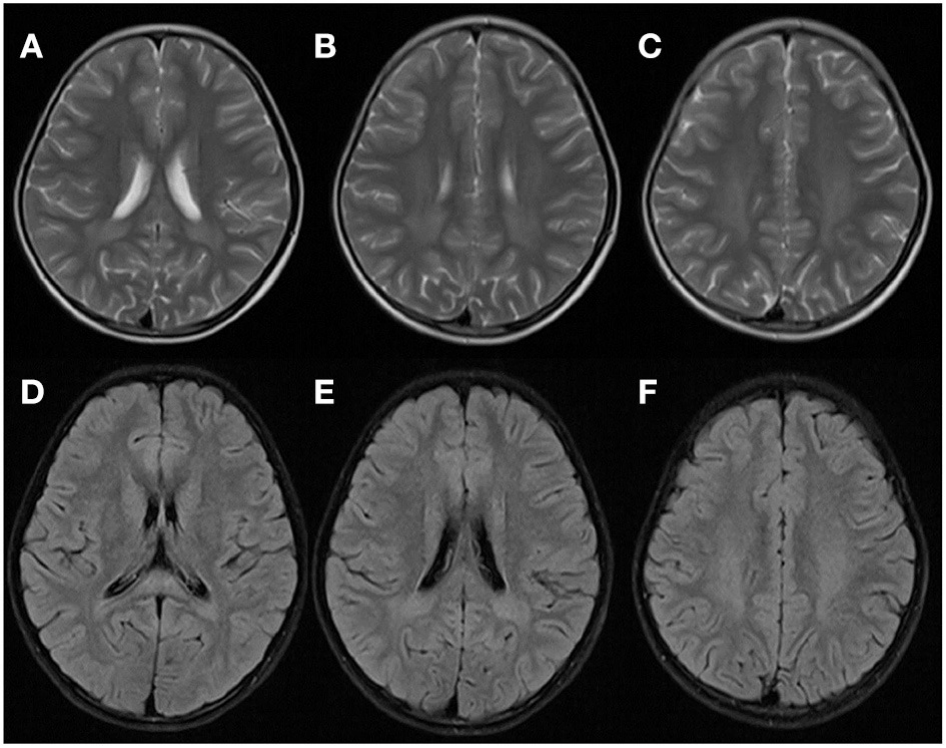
Brain MRI of the patient at the second episode of disease 2 years later. WM appeared hyperintense in T2-weighted axial (A–C) and FLAIR axial (D–F) views, which was similar with that observed during the first attack.
